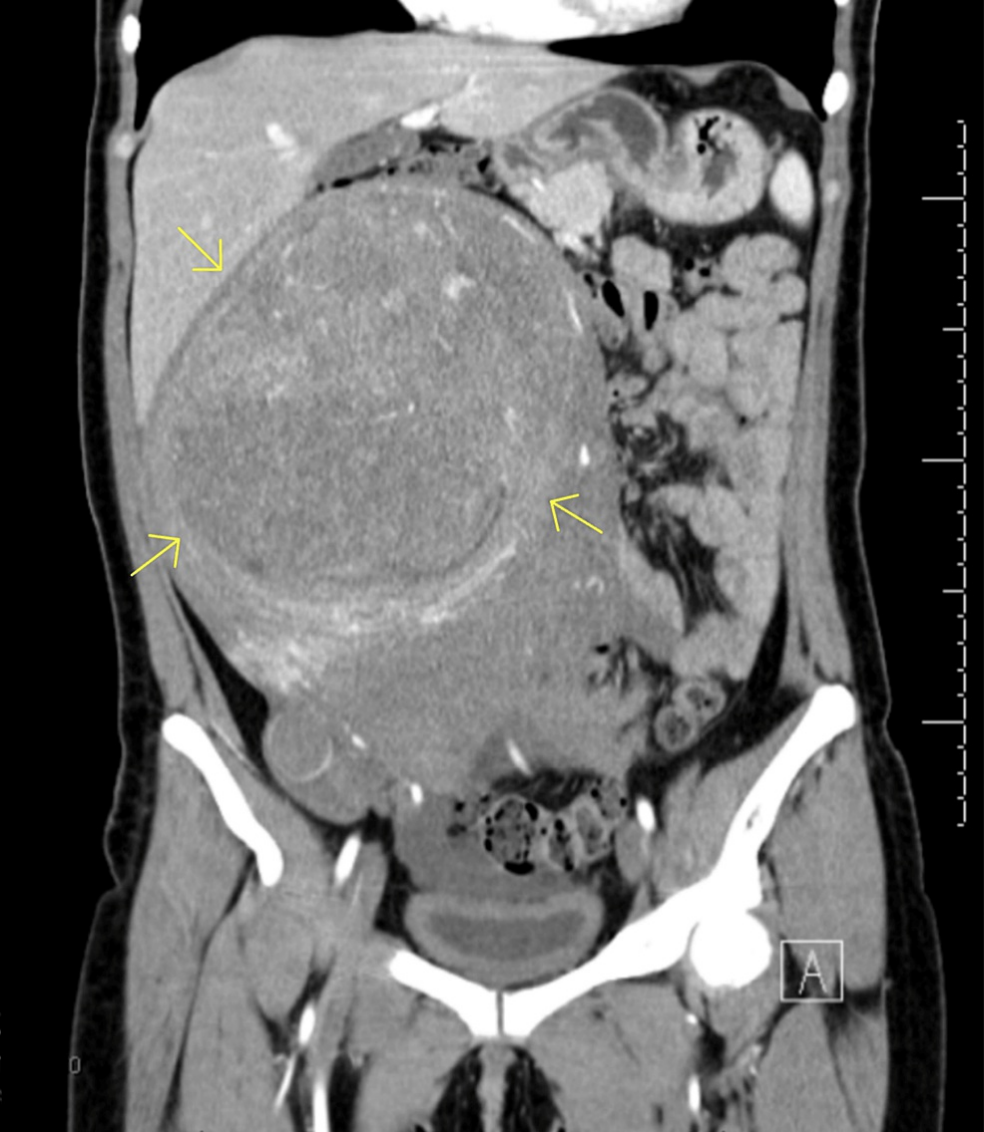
Computed tomography scan displaying a coronal view of the abdomen and pelvis exhibiting an intra-abdominal/pelvic mass (depicted by the yellow arrows) appearing to originate from the uterine fundus
